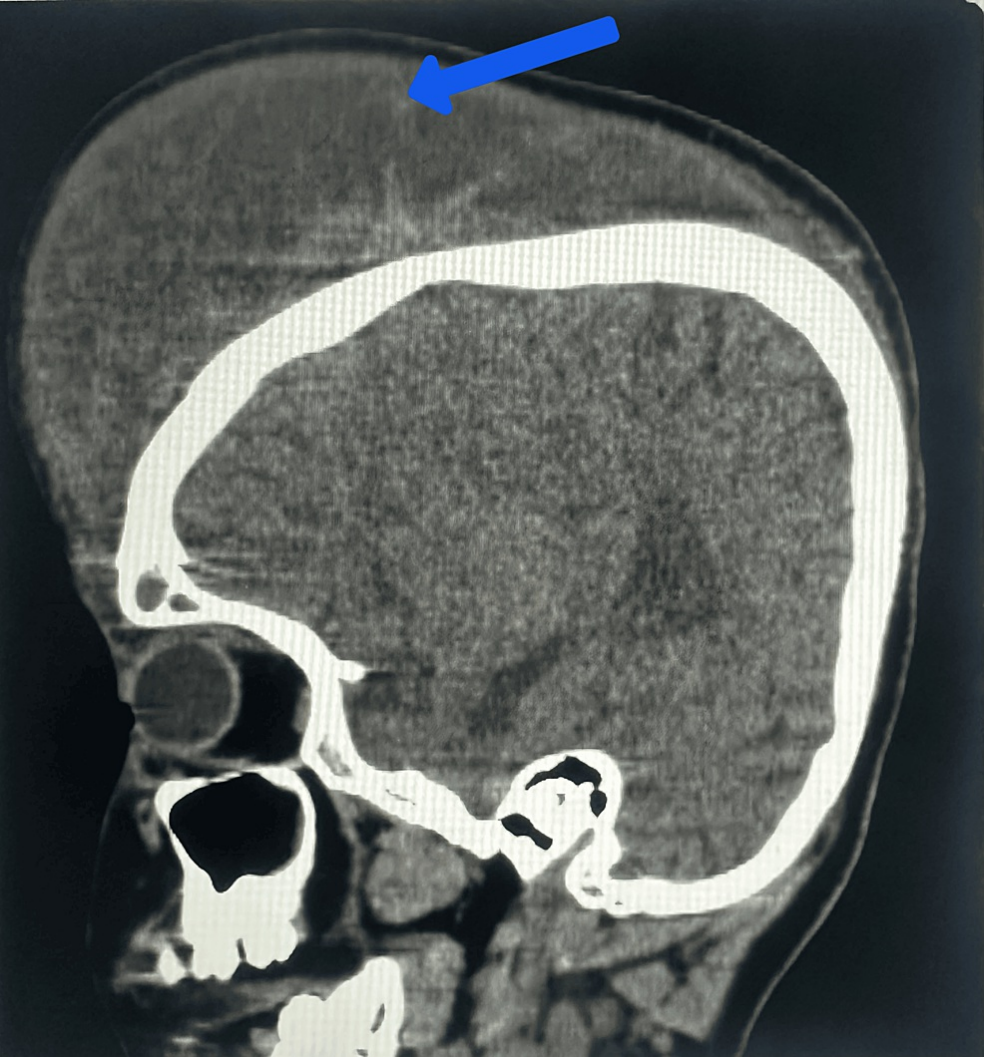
Non-contrast computed tomography, sagittal sections showing the extracalvarial collection of approximately 1300 cc in the frontoparietal region, as shown by the blue arrow
